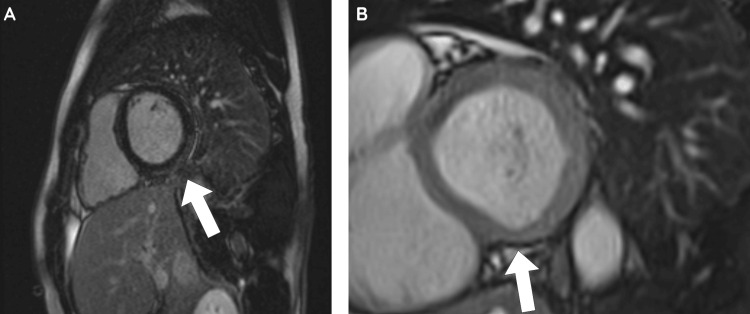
Cardiac Magnetic Resonance Imaging (CMRI), Late Gadolinium Enhancement (LGE) Imaging, and Short-Axis Cross-Section. Image A Shows Late Gadolinium Subepicardial Enhancement in the Basal Inferior and Inferolateral Segments (Highlighted by the Arrow). Image B Shows a Still Image of the Mild Hypokinesis of the Basal Inferior and Inferolateral Segments (Highlighted by the Arrow).
